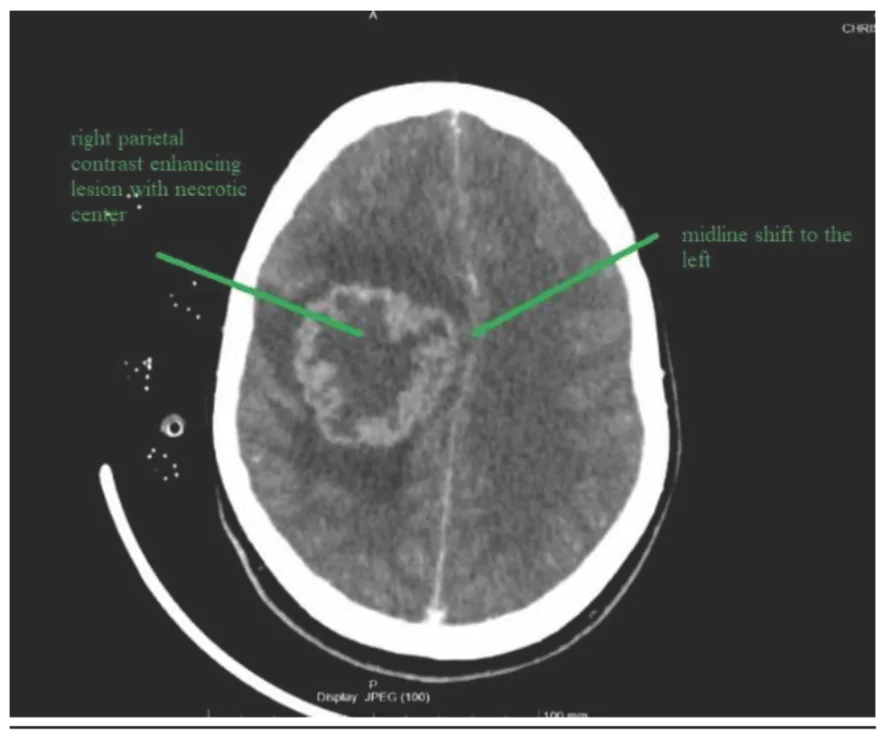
Axial CT scan showing a heterogeneously enhancing mass with central necrosis and surrounding edema in the right parietal region.
